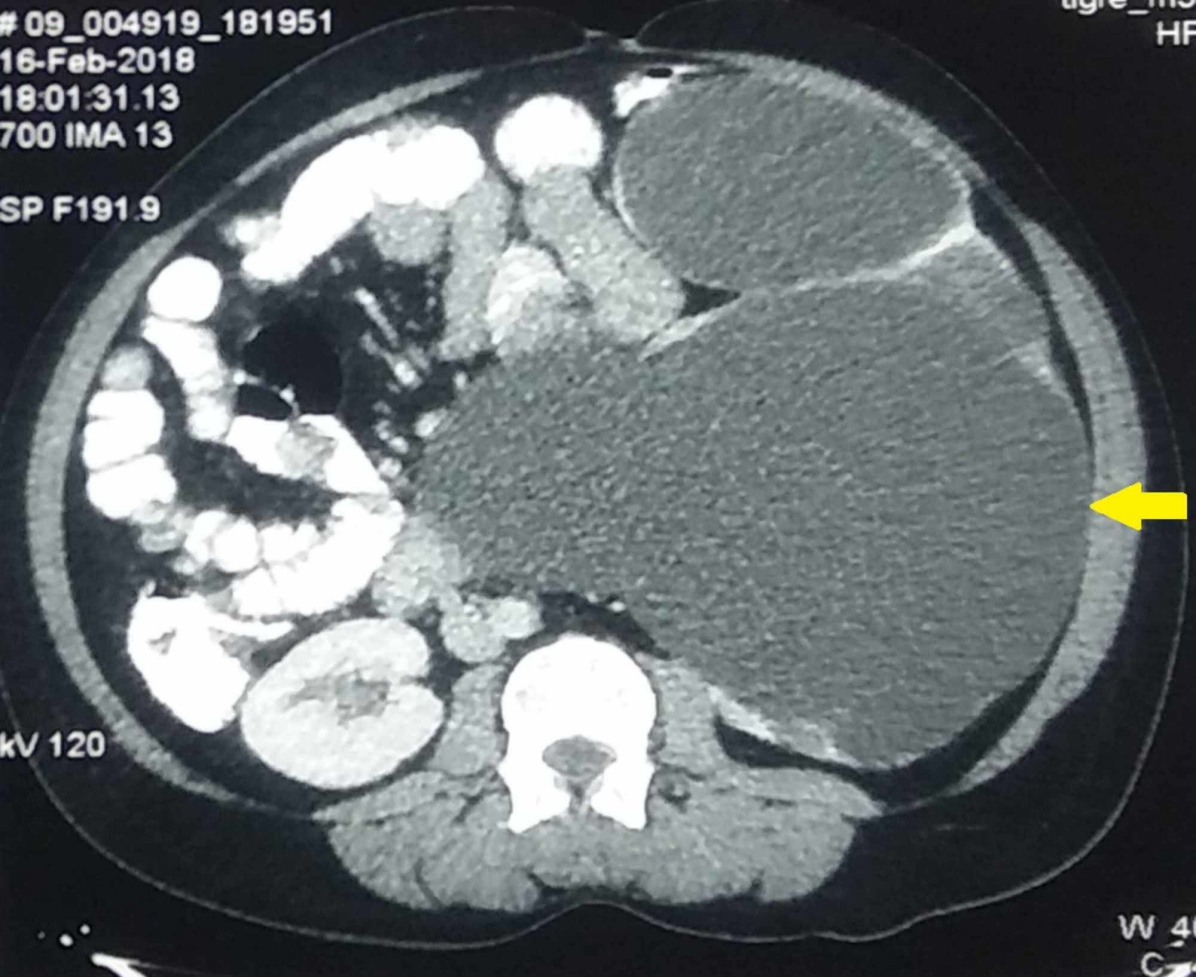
Contrast-enhanced computed tomography showing the giant hydronephrotic left kidney (arrow) without contrast excretion and the bowel being pushed to the contralateral side
